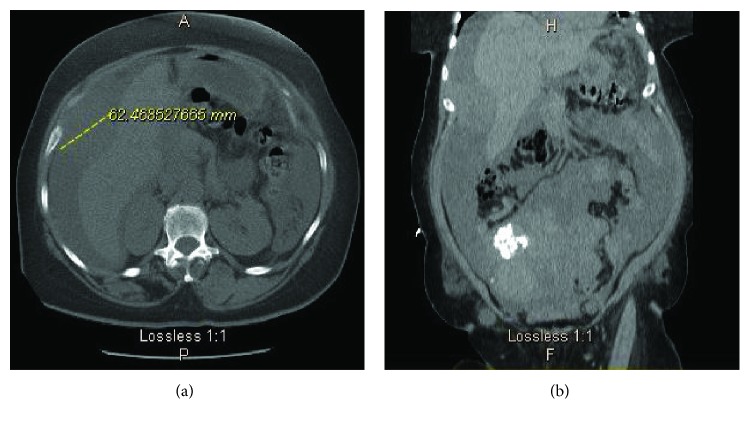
CT abdomen and pelvis without contrast showing a mass attached to the right lobe of the liver and peritoneal ascites. (a) Axial section. (b) Coronal section.
